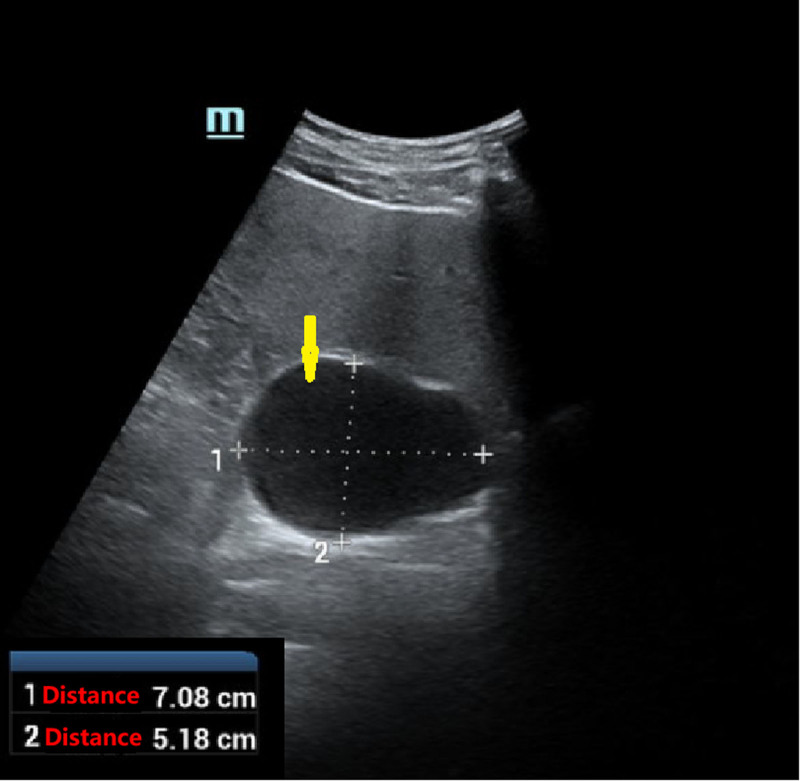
Ultrasound showed a cystic occupancy detected adjacent to the caudate lobe of the liver, suspected to be of hepatic origin, with a cyst measuring approximately 7.1 cm × 5.2 cm. the yellow arrow points to the cyst.
